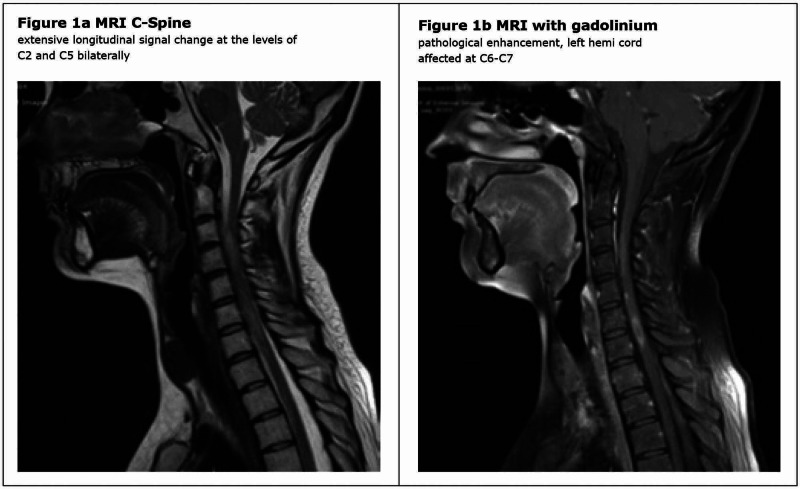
MRI cervical spine - four weeks post injury
